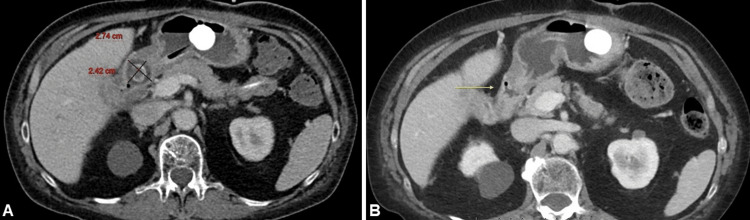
(A) Axial plane CT of the upper abdomen after lithotripsy, showing significant reduction in the gallstone size. (B) Final CT of the abdomen demonstrates passage of the stone and marked reduction in the periduodenal inflammatory changes (yellow arrow).
